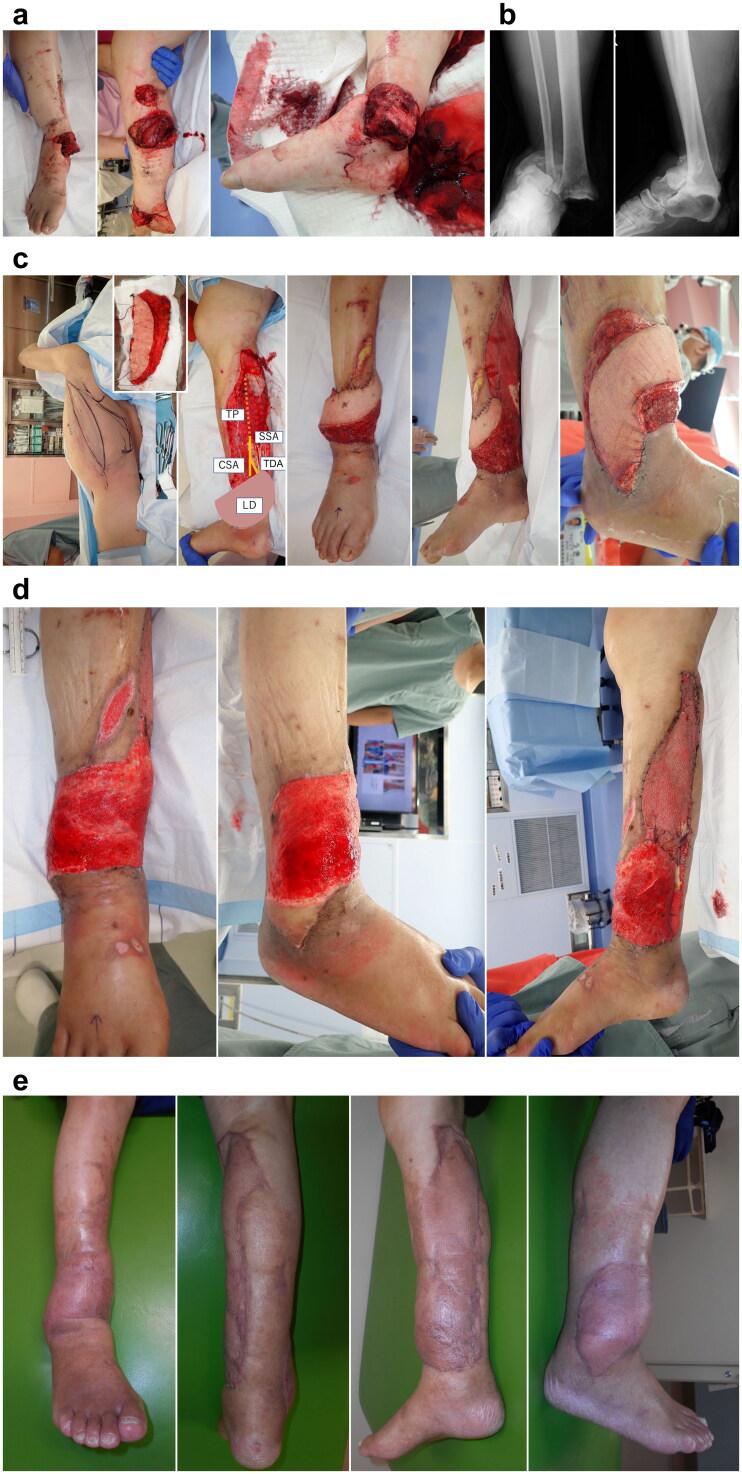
(A) Appearance on his arrival. (B) Radiographic findings show ankle dislocation fracture. (C) Free LD flap was performed at 4 weeks after injury. The recipient artery was interposed with T portion and the flow was sutured through anastomosis to the TP artery. (D) The fourth tangential excision was performed at 3.5 weeks after free LD flap. (E) Findings at 3 months after free LD flap. TP: tibialis posterior artery; SSA: subscapular artery; CSA: circumflex scapular artery; TDA: thoracodorsal artery; LD: latissimus dorsi; LD: latissimus dorsi TDA: thoracodorsal artery; UA: ulnar artery.
